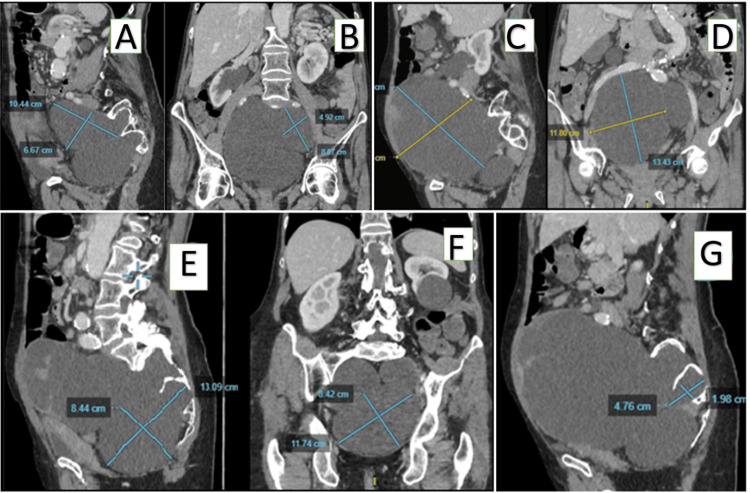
Preoperative abdominal CT scan. Sagittal (A) and coronal (B) imaging of the left S1 meningocele (11 x 5 x 5 cm), sagittal (C) and coronal (D) imaging of the right S1 meningocele (12 x 15 x 16 cm), sagittal (E) and coronal (F) imaging of the left S2 (10 x 11 x 8 cm), and sagittal imaging of the right S2 meningocele (G) (3.7 x 3.5 x 3.2 cm). Meningoceles described are outlined with white arrows.
